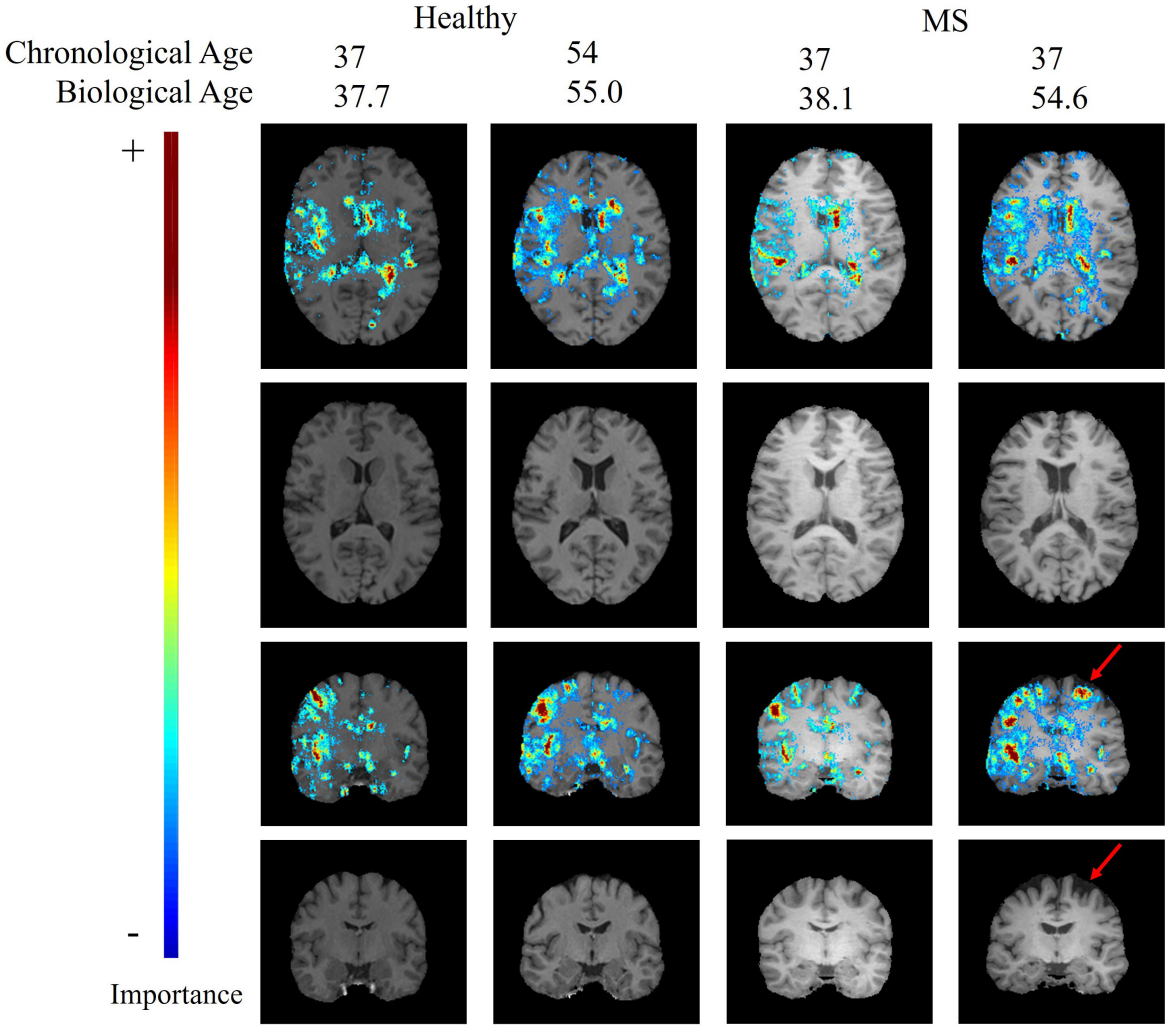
Age-specific T1-weighted MRI with and without saliency maps from an axial and a coronal view of two healthy subjects and two MS patients. The red arrow indicates a patient-specific region with brain atrophy caused by neurodegeneration.
